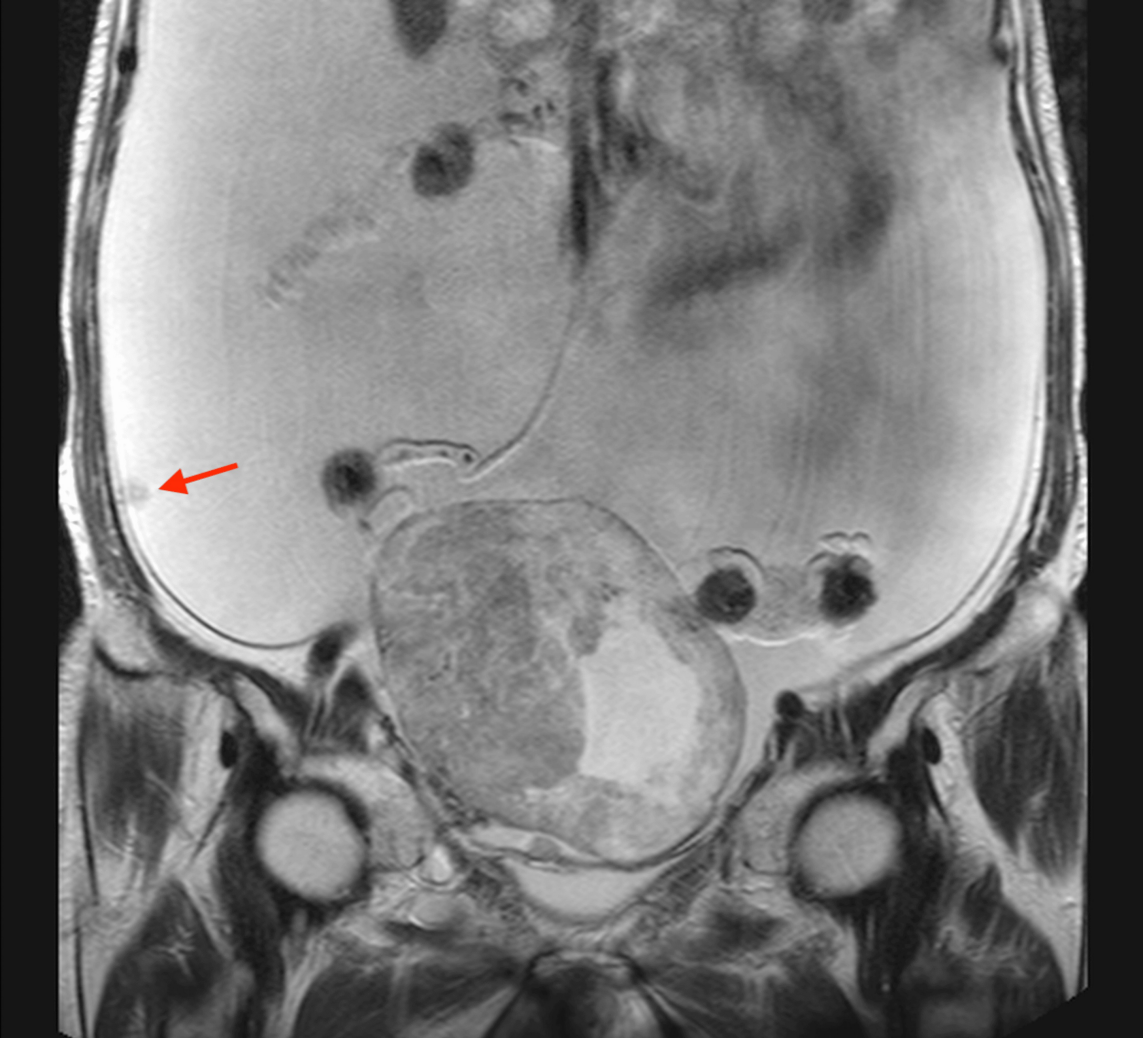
This is the same patient as shown in Figure 1. The coronal T2-weighted image reveals massive ascites and peritoneal dissemination (arrow).
